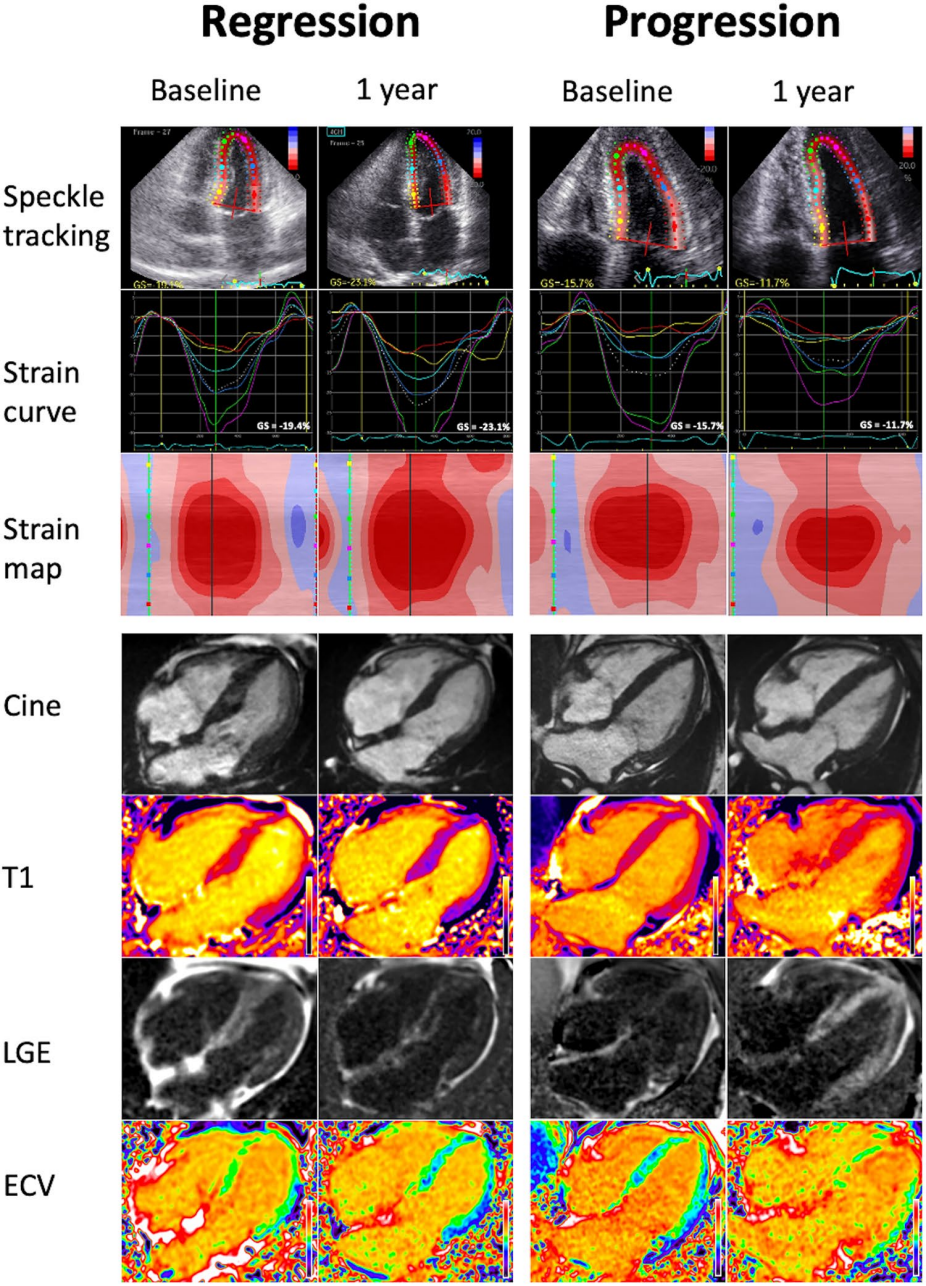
Top panel: echocardiographic global strain (GS) measurements demonstrating an improvement in a patient with a good haematological response to chemotherapy, and a deterioration in another patient with a poor haematological response. Bottom panel: cardiac magnetic resonance imaging demonstrating a reduction in native T1, late gadolinium enhancement (LGE) and extracellular volume (ECV) in a patient with a good haematological response to chemotherapy, and a deterioration in another patient with a poor haematological response
